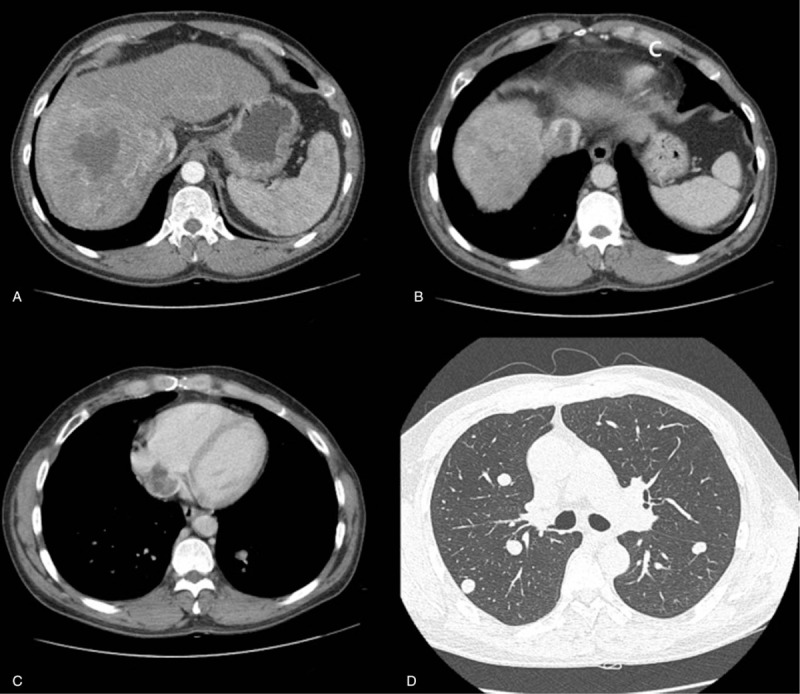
Initial abdominal computed tomography (CT) revealed a 12.6 × 12.2-cm-sized hepatocellular carcinoma with daughter nodules in the right hepatic lobe (A), tumor thrombosis in the suprahepatic inferior vena cava (B), and metastasis to the right atrium (C). Chest CT showed multiple well-defined nodules in both lungs, suggesting pulmonary metastasis (D).
